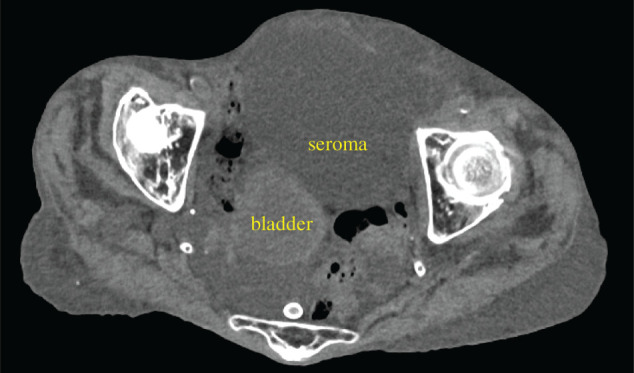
CT on POD 23 demonstrating a large postoperative seroma (approximately 12 × 12 cm) in the left preperitoneal space, compressing the urinary bladder.
